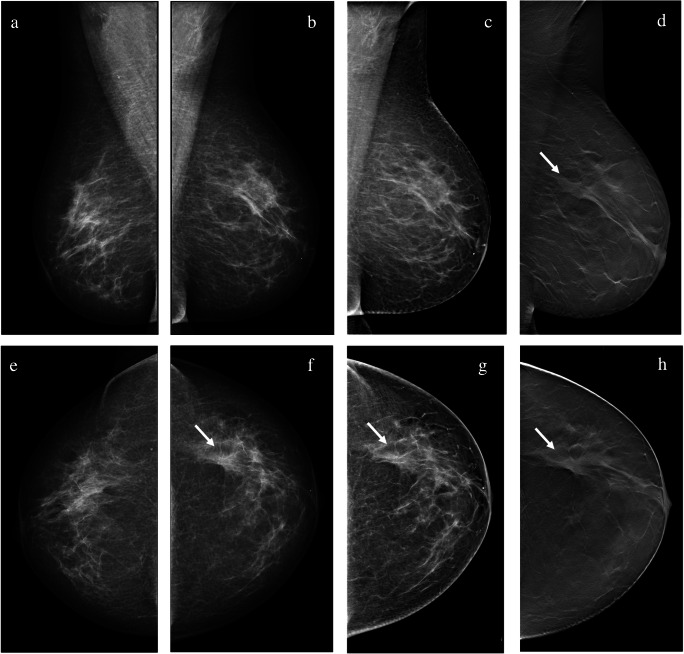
A 56-year-old woman with suspicious calcifications on the right side (benign at biopsy). An architectural distortion was visible in the upper outer quadrant of the left breast (arrow). The lesion was detected and correctly classified as suspicious by one in four readers with digital mammography (DM) and with one-view wide-angle digital breast tomosynthesis with synthetic mammography (WA-DBT with SM), while it was detected and classified as suspicious by three in four readers with two-view WA-DBT with SM. The lesion was biopsied under ultrasound guidance, and the diagnosis was invasive ductal carcinoma grade 2 with ductal carcinoma in situ (a, b DM bilateral mediolateral oblique view (MLO); c SM MLO; d DBT MLO; e, f DM bilateral cranio-caudal view (CC); g SM CC; h DBT CC)
